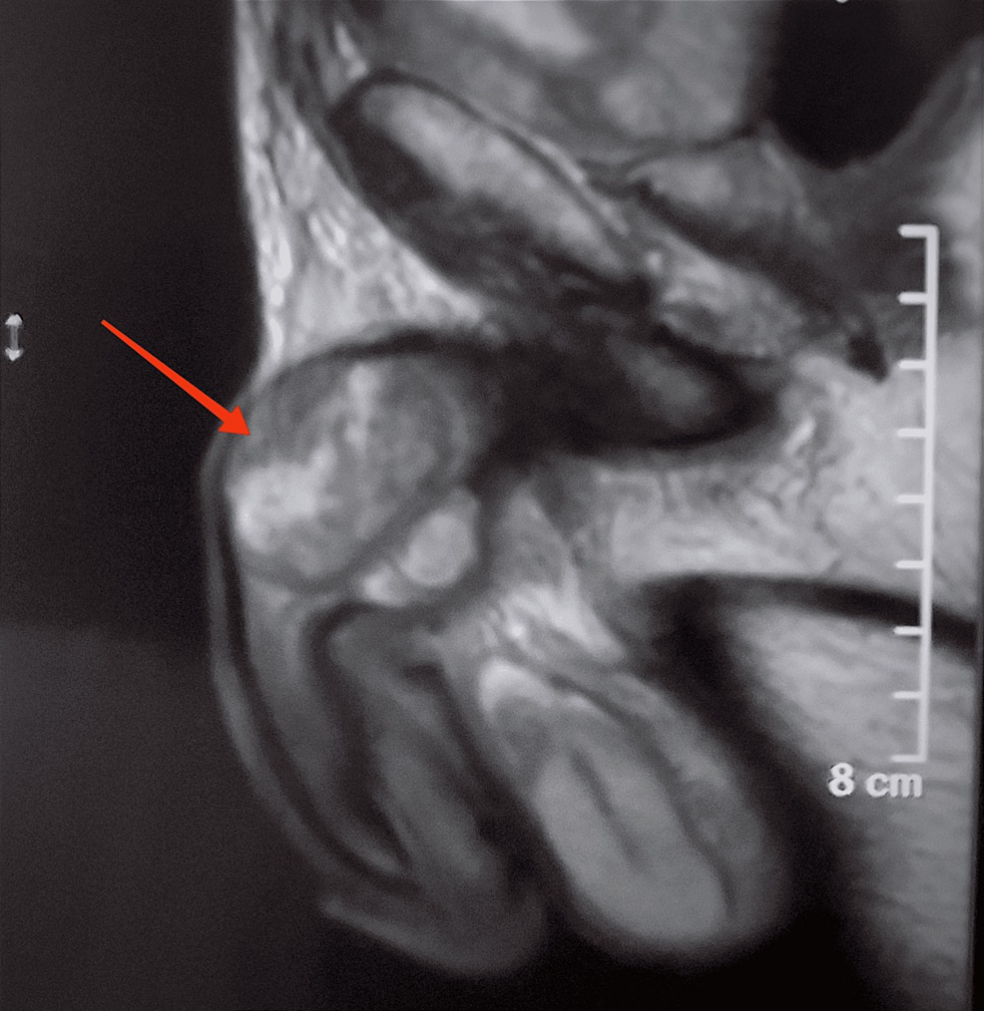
Pelvic MRI showing a nodular aspect infiltrating the corpora cavernosa without involvement of the corpus spongiosum or the urethra, with a tumoral appearance.
